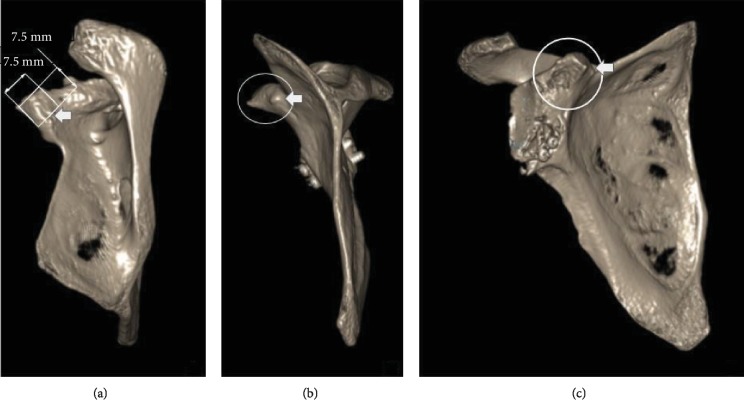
CT scan showing the coracoid process remnant's measure. (a) Scapula superior view, measure from the base to the distal end of the previous osteotomy; total length and width was 7.5 mm. (b) Scapula medial view. (c) Scapula anterior view.
